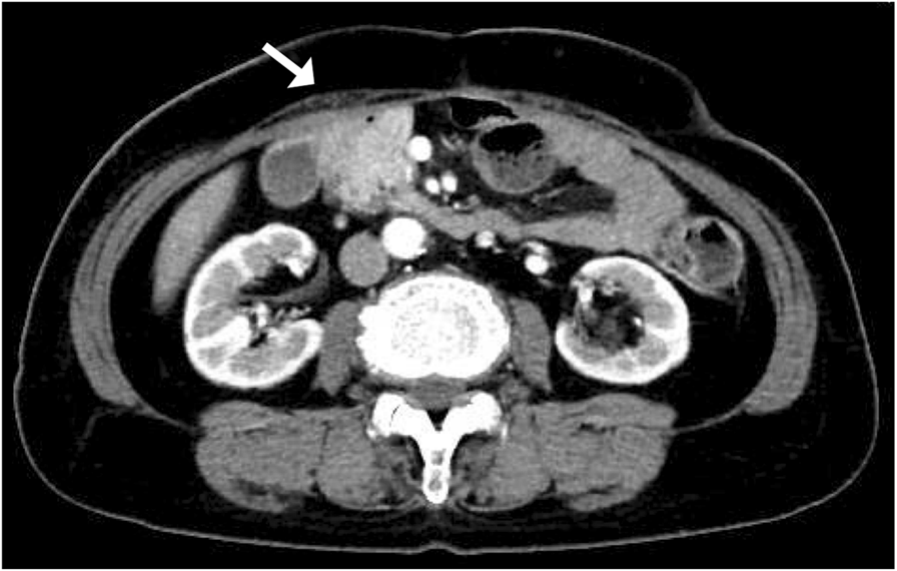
Contrast-enhanced abdominal CT after chemotherapy showed reduced duodenum tumor and abdominal wall thickness (arrow)
